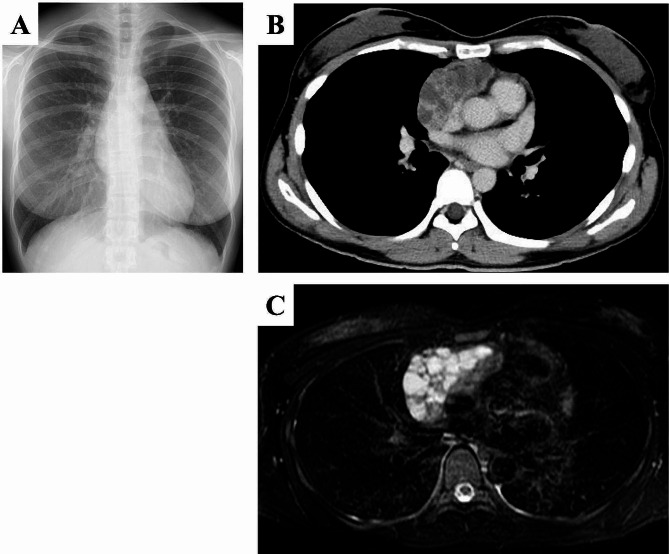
Imaging findings of thymic mucosa-associated lymphoid tissue. A chest radiograph shows a tumor with a positive silhouette sign in the right atrium. There is no evidence of cardiomegaly (A). Chest computed tomography shows a 9.0 cm mass consisting of a heterogeneous multifocal cyst and a contrast-effective septate in the anterior mediastinum (B). T2-weighted magnetic resonance imaging of the chest shows a lobulated mass with cyst-like high-signal clusters. The tumor does not invade the surrounding organs (C)
